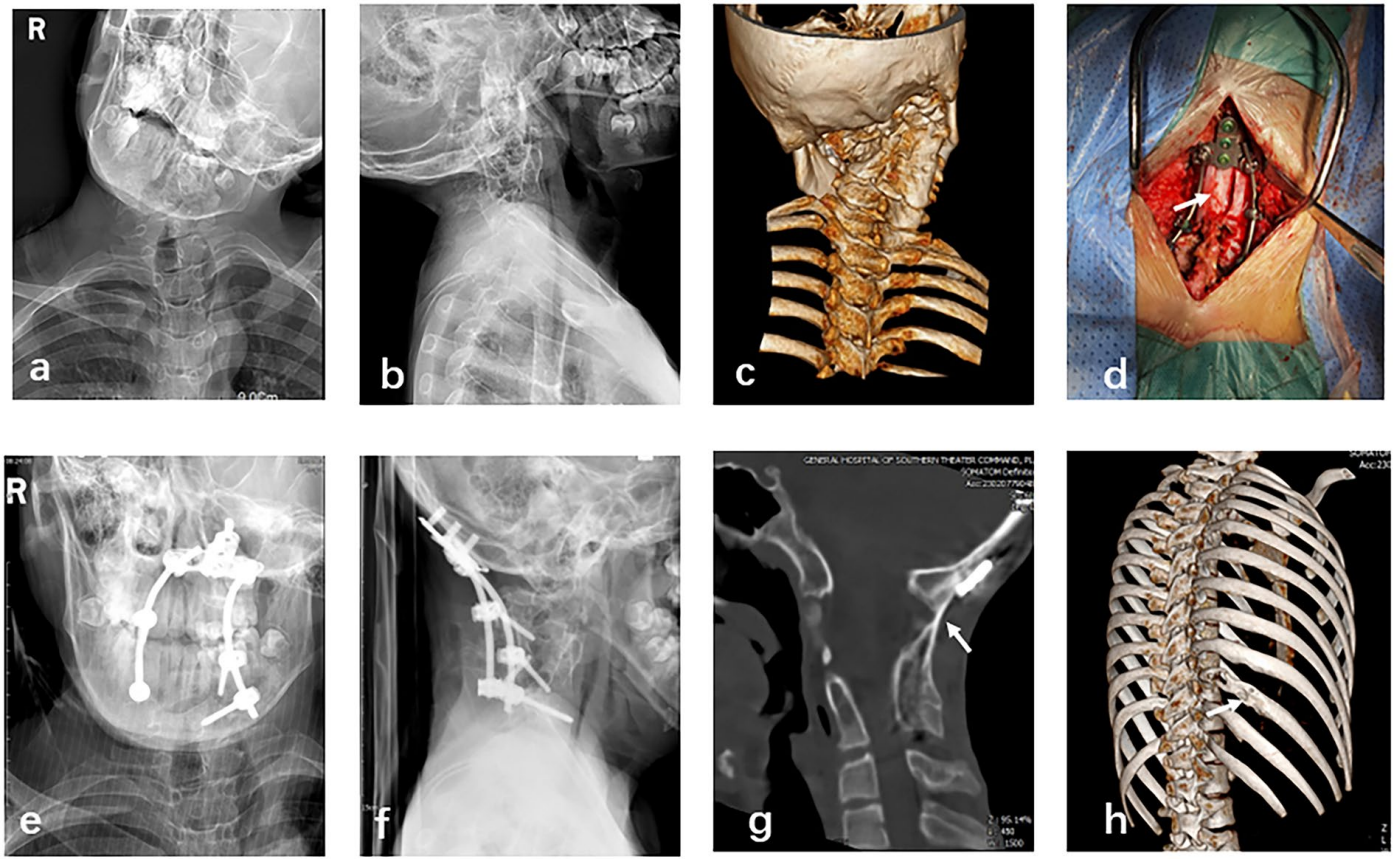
A 13-year-old male diagnosed with KFS. a–c: Preoperative X-ray and CT 3D reconstruction showing cervical deformities and partial vertebral bone fusion. d: Intraoperative application of C0–C6 internal fixation and autologous rib bone grafting. e, f: Postoperative X-rays of the cervical spine (sagittal and lateral views) showing good positioning of the internal fixation. g: Follow-up cervical spine CT at 6 months after surgery showing good bone fusion at the graft site. h: Chest CT 3D reconstruction at 6 months after surgery showing partial regeneration of the rib defect at the donor site
